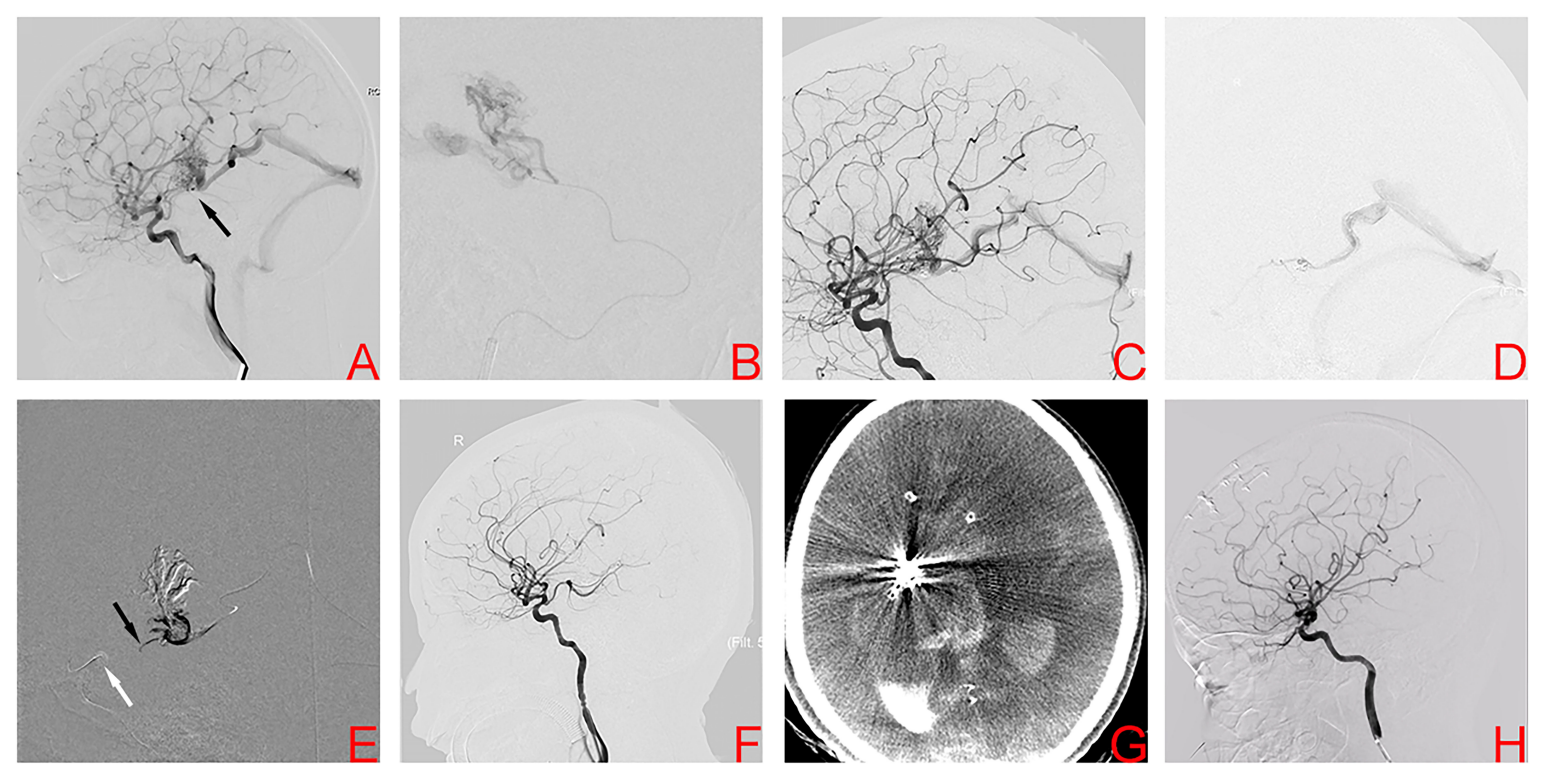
Digital subtraction angiography demonstrates the right basal ganglia arteriovenous malformation in case 2 (A). Superselective arteriography and embolization *via* the posterior communicating artery (B). Angiography after transarterial embolization shows a partially embolized arteriovenous malformation (C). TVE was performed due to the lack of optimal arterial access. Transvenous microcatheter injection angiography confirmed an optimal position of the microcatheter tip (D). After the balloon (white arrow) was inflated to provisionally occlude the ipsilateral internal carotid artery, Onyx was injected transvenously into the AVM nidus through the microcatheter, and ~2 cm of embolysate reflux (black arrow) was encountered prior to achieving sufficient retrograde nidal penetration (E). Postoperative angiography showed complete embolization (F). However, postoperative CT confirmed intracranial hemorrhage (G). Angiography 1 year later showed complete embolization of the arteriovenous malformation (H).
